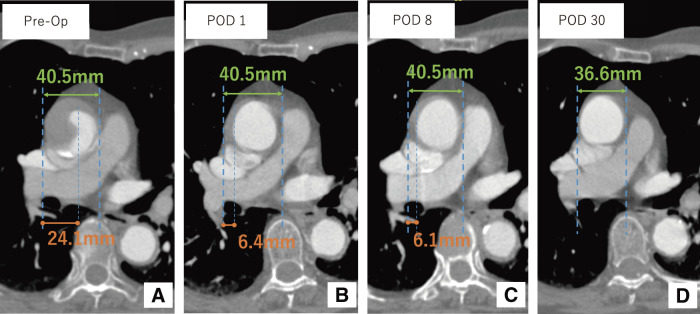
Fig. 3 Axial contrast-enhanced CT images obtained preoperatively (single image) and postoperatively (multiple images). (A) Pre-treatment image shows true lumen compression and contrast pooling in the false lumen. The superior vena cava is compressed by the enlarged aorta. (B) On POD 1, the true lumen is expanded and the false lumen thickness is reduced. (C) The false lumen thickness continues to decrease. (D) By POD 30, the false lumen is no longer visible and the aortic diameter has decreased. Op: operation; POD: postoperative day
